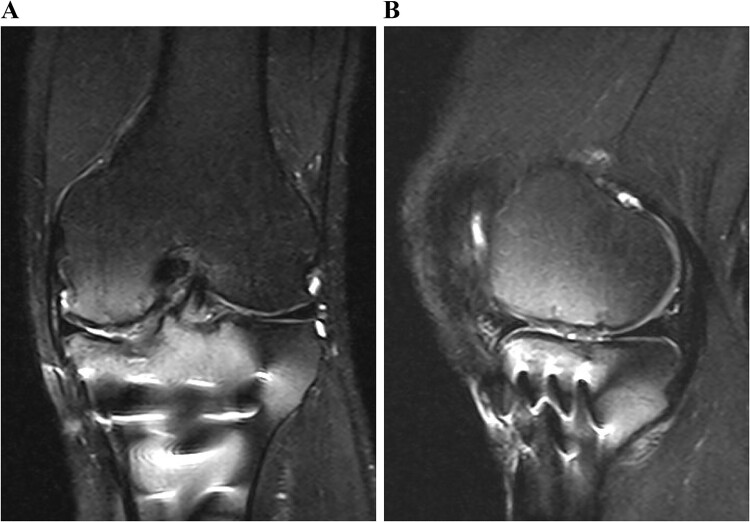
Postoperative T2-weighted fat-suppressed magnetic resonance images at 1 year after surgery. The transplanted cultured cartilage was successfully integrated without any delamination.
